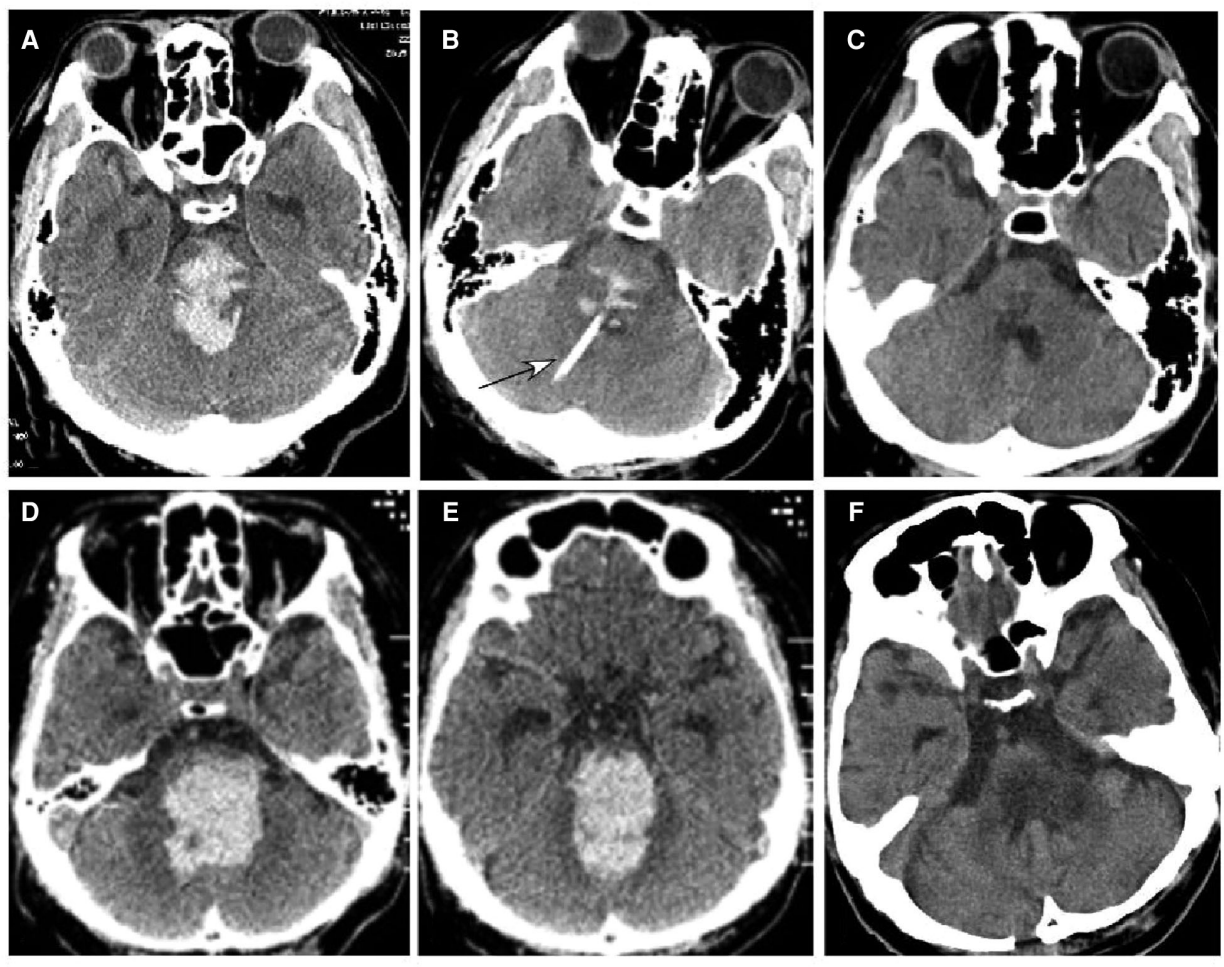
Brainstem hemorrhage. (A–C) A 48-year-old man had a sudden loss of consciousness 6 h before presentation. He was in a coma with a Glasgow Coma Scale (GCS) score of 3 (E1VTM2) but no spontaneous breathing before surgery. Computed tomography (CT) revealed brainstem hemorrhage (A). Stereotactic aspiration was performed (B, arrow). One month after surgery (C), the hemorrhage disappeared, and he regained his spontaneous breathing and could open his eyes with the GCS of E4TM5. (D–F) A 48-year-old man had a sudden onset of unconsciousness 6 h before being transferred to our hospital. CT scan showed severe hemorrhage in the brainstem and the fourth lateral ventricle (D,E), and he was in a coma with a GCS score of 3 (E1VTM2) but no spontaneous breathing. Preoperative estimation was that he might not survive, but his family was strongly in favor of surgical treatment. One week after the stereotactic aspiration (F), he could open his eyes, and on the ninth day, the hemorrhage disappeared completely.
